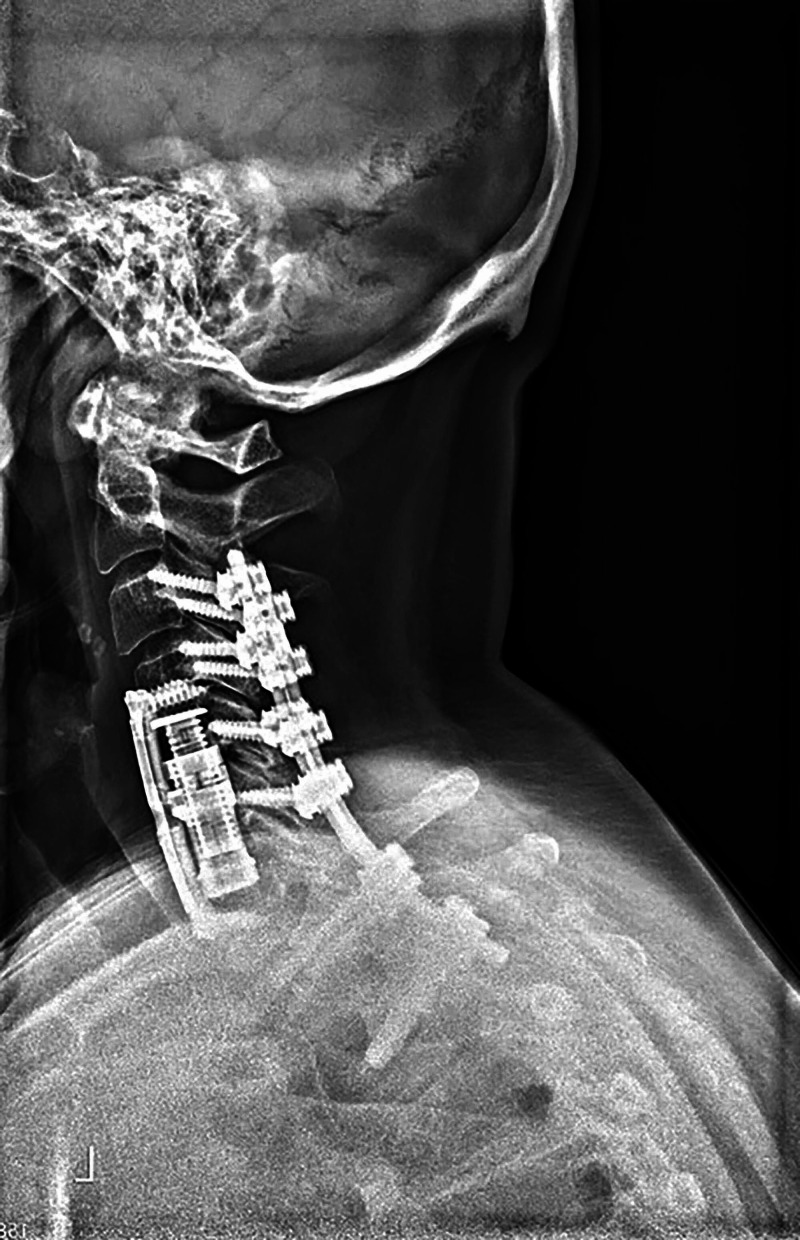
Postoperative lateral standing x-ray showing the anterior and posterior stabilization construct.
